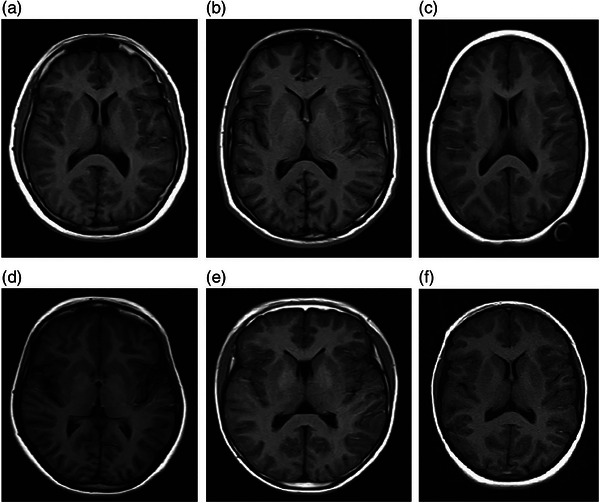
Axial T1/SE magnetic resonance imaging (MRI) view of (a) a 25‐year‐old adult male with Down syndrome (DS), (b) a 25‐year‐old male control participant, (c) a 12‐year‐old female with DS, (d) a 12‐year‐old female control participant, (e) a 14‐year‐old male with DS, and (f) a 14‐year‐old female with DS for comparison between DS and control participants as well as between DS males and females.
